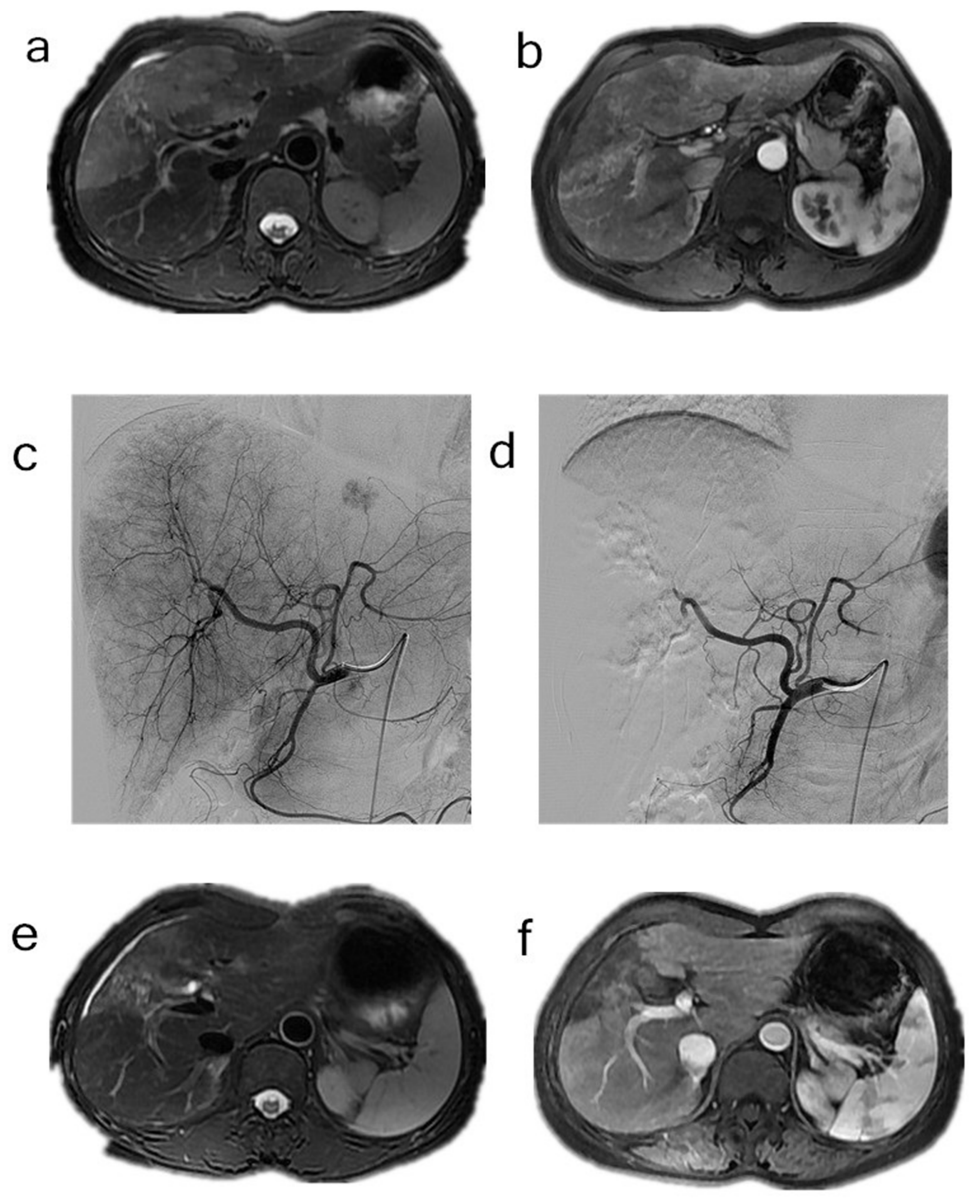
a-b. Contrast-enhanced MRI and CT scan done before the procedure in the patient with cholangiocarcinoma, c. Common hepatic artery angiography of the patient with cholangiocarcinoma, d. After chemoembolization angiography with extraction of pathologic vascularization, e-f. Gadolinium contrast MRI control 6 months after TACE, extraction of the vascularization of the tumor.
